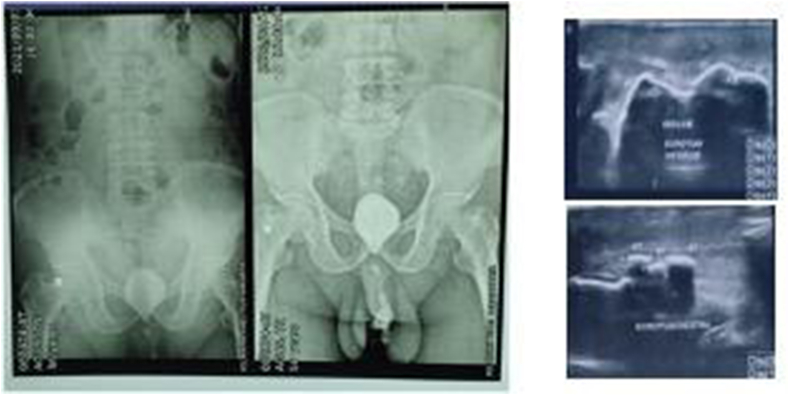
Abdominal X-ray and scrotal ultrasound showed suggestive of vesicolithiasis and multiple urethral stones.
